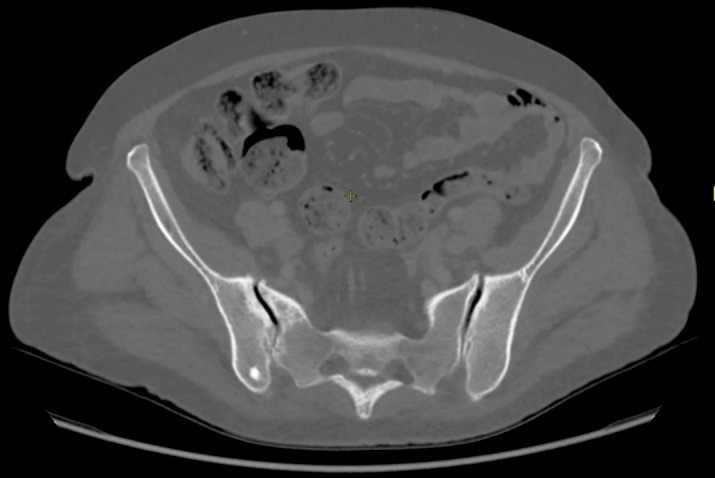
Sacro-iliite droite. Sclérose sous-chondrale et erosions sur le côté iliaque et sacré de l'articulation. Ostéophytes marginaux. Scanner Right sacroiliitis. Subchondral sclerosis and erosions on the iliac and sacral side of the joint. Marginal osteophytes. CT Scan
